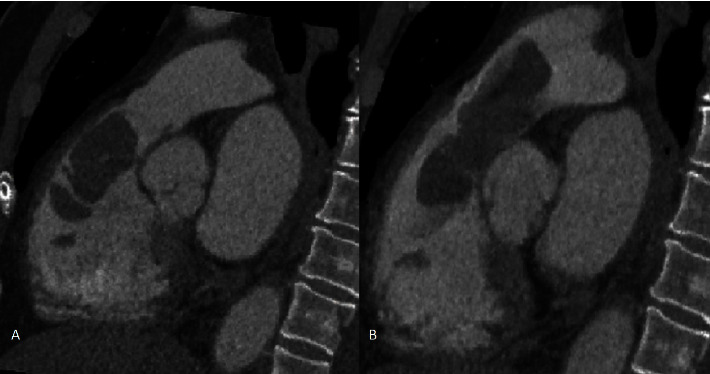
Gated computed tomographic angiography of the chest showing the mass in the RV (a) traversing the pulmonic valve into the main pulmonary artery. (b) Abbreviations: RV: right ventricle.
